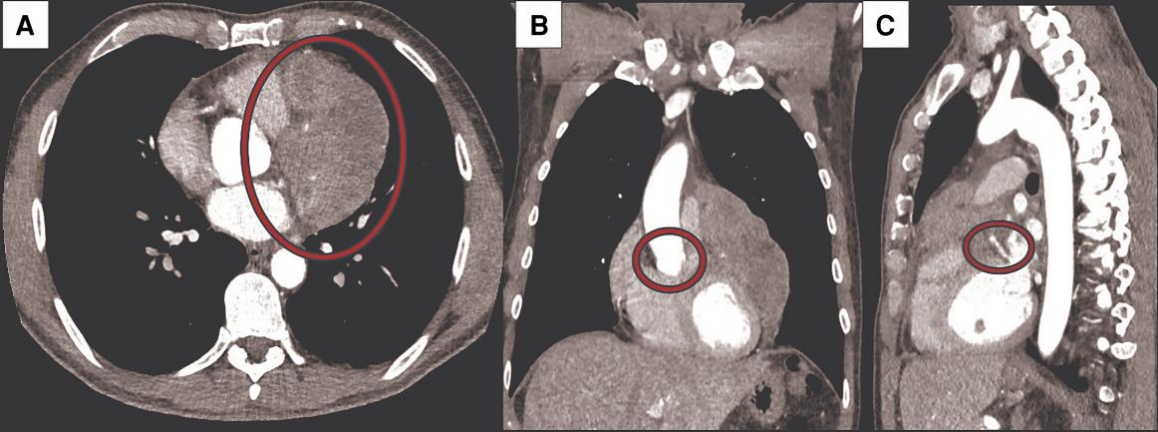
Computed tomography images of the pericardial mass. The mass has an inhomogeneous surface involving the anterolateral wall of the left ventricle (*A*), the right ventricle outflow tract (*B*), and encircling the left anterior descending coronary artery (*C*).
